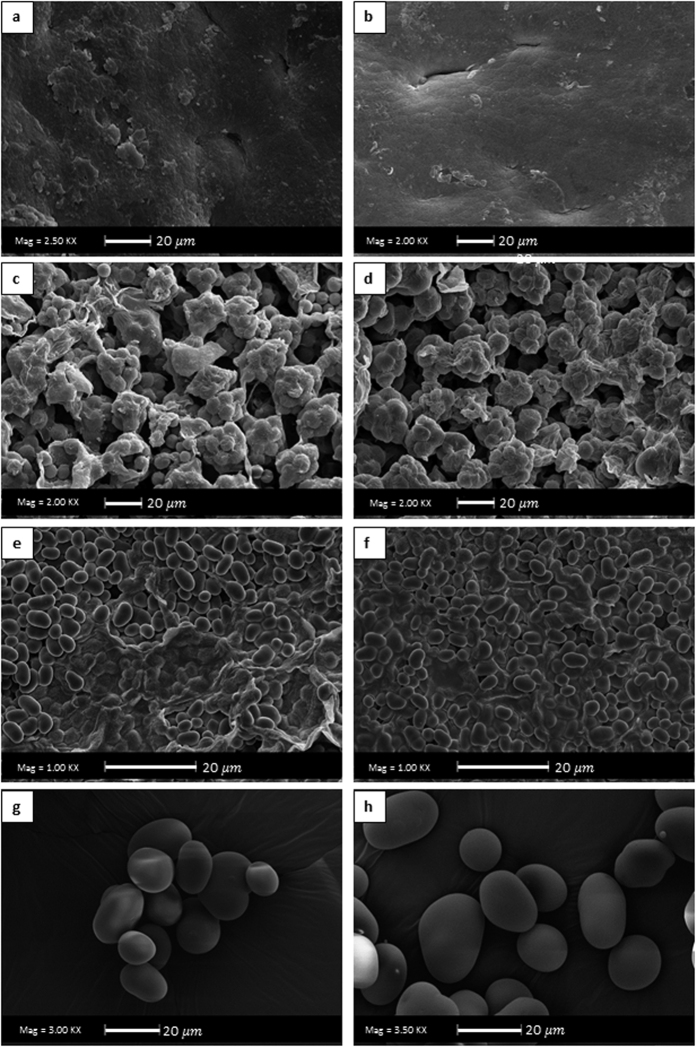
Microphotography SEM (20 kV; the magnifications are shown in the figures) of the different structures and starch of mung bean. (a,c,e and g) are the seed coat surface, hilum, cotyledon and starch, respectively, from conventionally hydrated beans. (b,d,f and h) are the seed coat surface, hilum, cotyledon and starch, respectively, from from ultrasound assisted hydrated beans.
